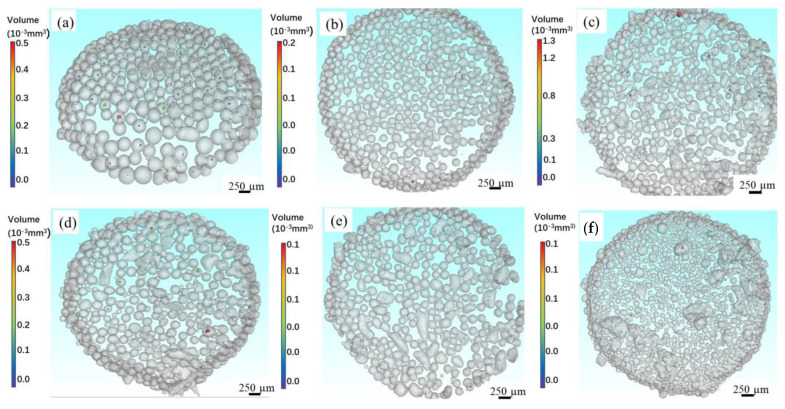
The 3D reconstructed CT of (a) Ti-6Al-4V, (b) Co-Cr-Mo, (c) 316L at 8000 RPM; as well as (d) Ti-6Al-4V, (e) Co-Cr-Mo, (f) 316-steel at 12,000 RPM.
